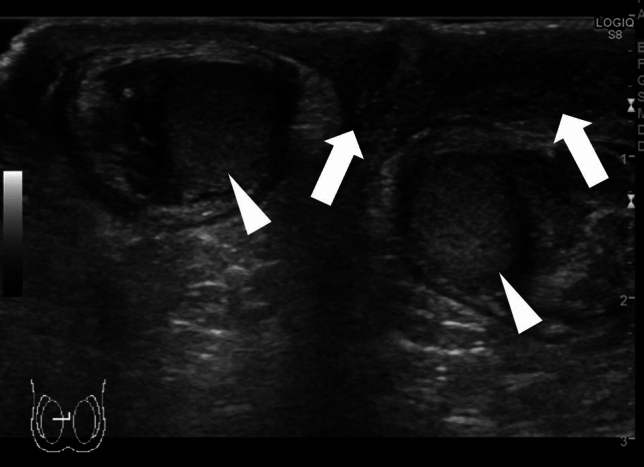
Ultrasonographic images of a 5-year-old boy with acute scrotum associated with IgA vasculitis. Edematous changes are evident in the dermis of the right and left scrota. Purpura was detected, leading to the diagnosis of IgA vasculitis
